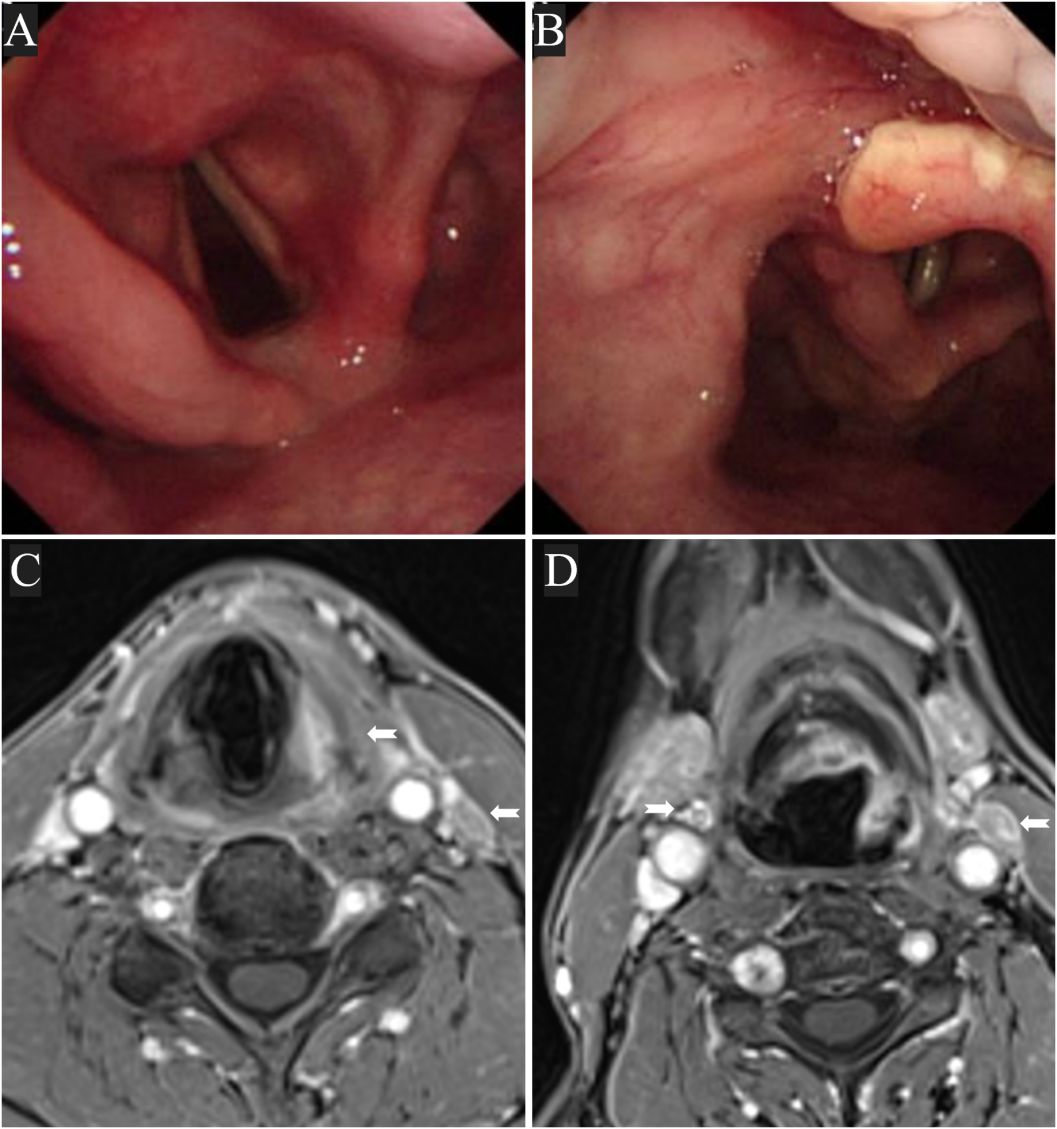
Laryngoscopy (A, B) and MRI (C, D) after neoadjuvant therapy. There was a marked tumor reduction (71.6% reduction in tumor volume), with no visible residual tumor on endoscopy. White arrows indicate areas of residual signal abnormality on MRI.
